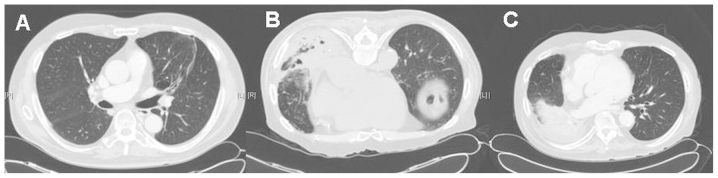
Case 2: (A) CT scan showing the lung after surgery, (B) CT scan three years after surgery showing recurrence of lung cancer and (C) CT scan after three cycles of chemotherapy revealed disease progression. CT, computed tomography.
